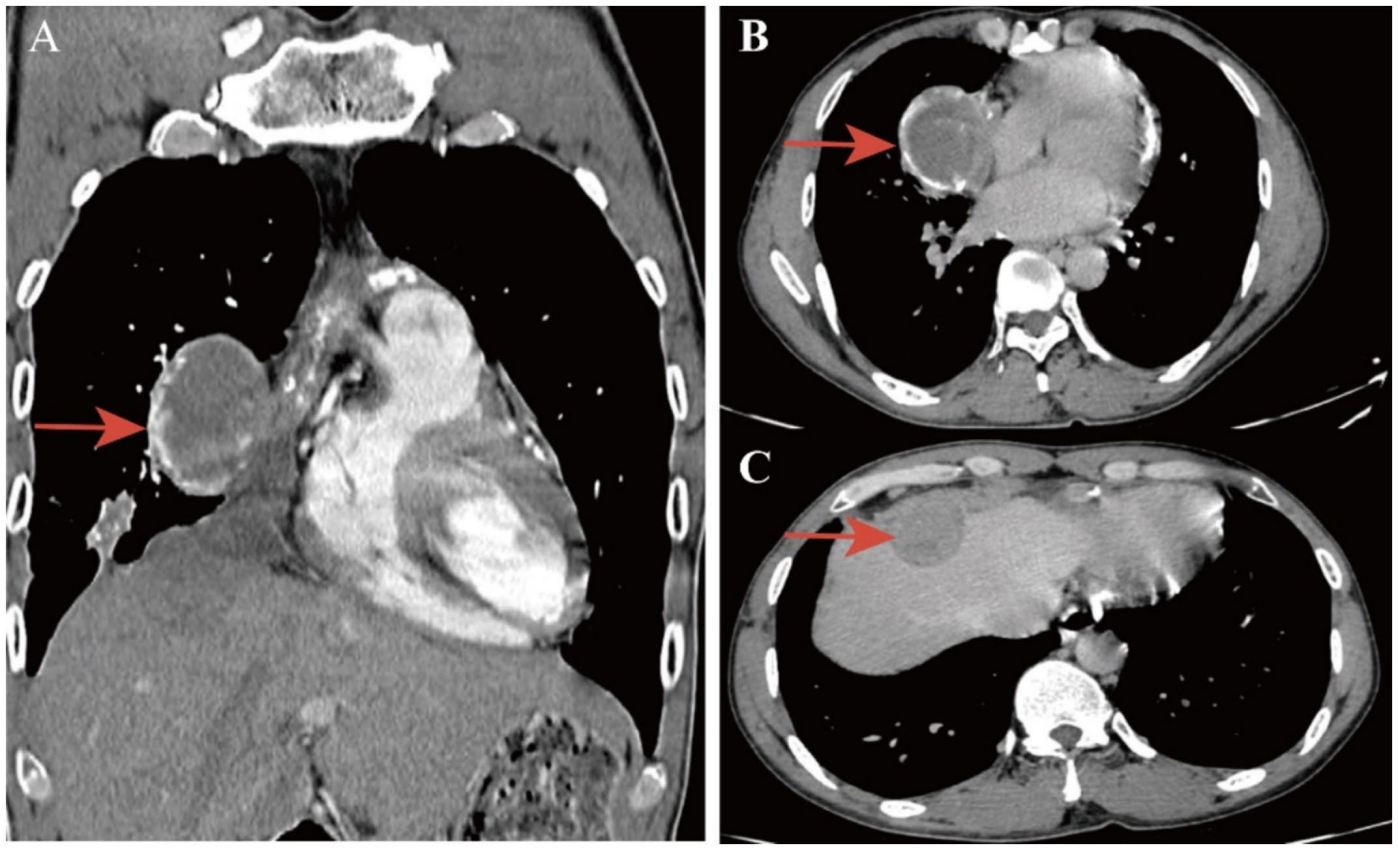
Enhanced chest CT showing lesions in the right mediastinal paravertebral and right cardiophrenic angle regions [(A) Coronal section, (B,C) Transverse section. Red arrows indicate mediastinal and liver capsule abscesses].
